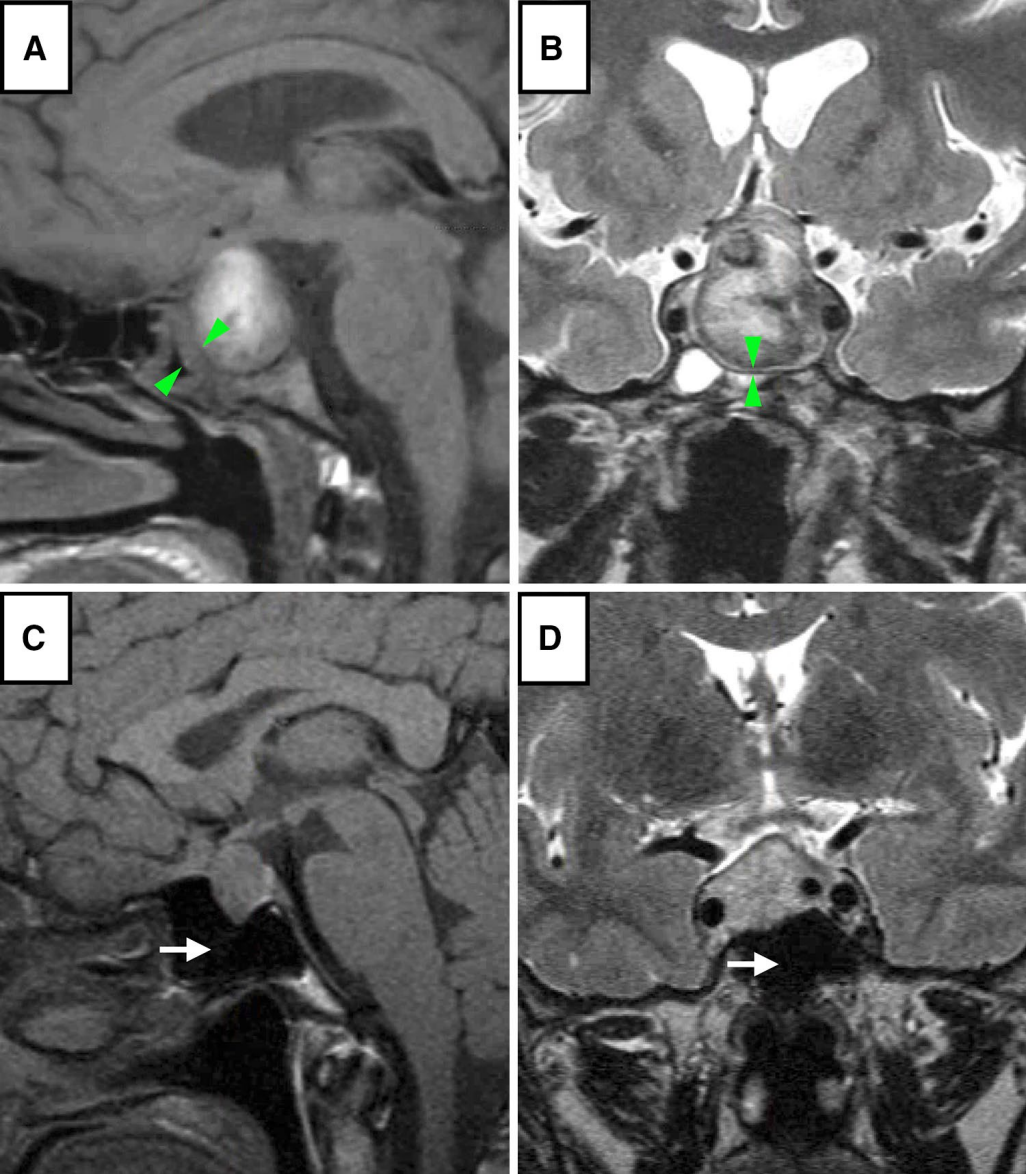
Sagittal T1 (a and c) and coronal T2-weighted (b and d) MRI from a patient presenting with PA (a and b) and NFPA (c and d). The patient with PA (a and b; Case A1, Table 1) was a 24 year old male, with no significant past medical history, who presented with acute confusion, headaches and hyponatraemia. MRI revealed a pituitary lesion with evidence of bleed and sphenoid sinus mucosal thickening (*green arrows*). The patient with NFPA (c and d; Case N3, Table 1) was a 54 year old female who presented with a homonymous hemianopia. MRI revealed a pituitary lesion abutting the optic chiasm and with no evidence of sphenoid sinus mucosal thickening and a relatively empty sinus (*white arrows*)
